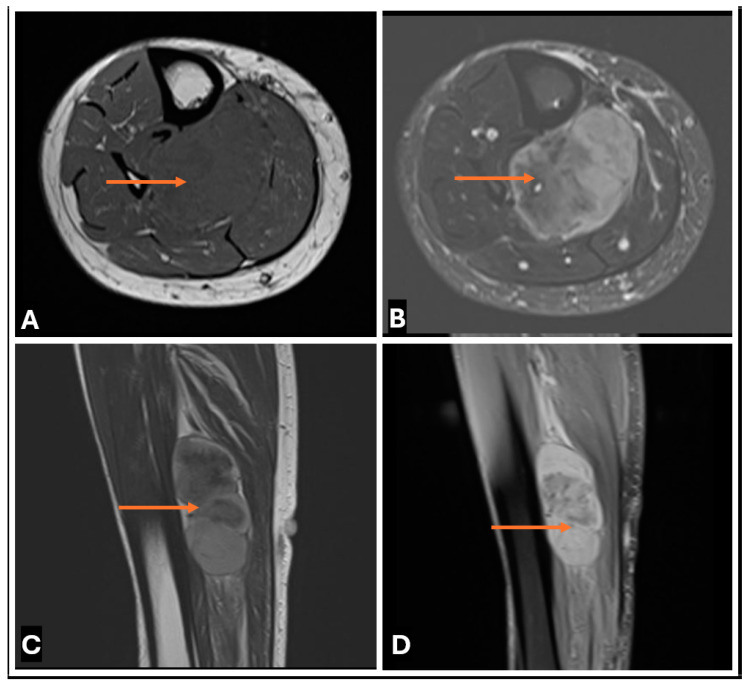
Primary Synovial sarcoma of the tibial nerve in a 30 year old female. (A–D) (A) T1-w Axial; (B) T2-w FS Axial; (C) T1-w Sagittal (D) T2-w FS Sagittal images showing Synovial sarcoma of the tibial nerve within the mid-lower leg as a lobulated heterogenous mass (thin arrows), with low to intermediate T1 signal and high T2 signal on MRI.
